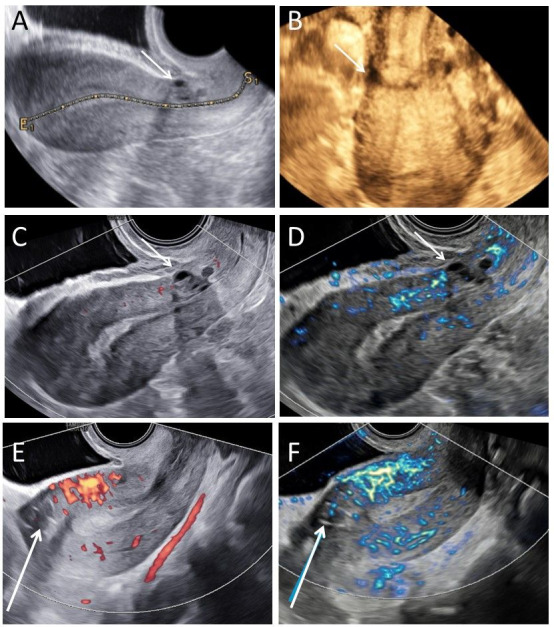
Representative images of pre- and postoperative assessment of scar tissue. A. Representative images of a complex niche shown in B-mode, sagittal view; B. Complex niche shown in three-Dimensional, coronal view; C. Complex niche shown in power Doppler mode; D. Complex niche shown in MV-flow^TM^ mode. Arrow indicates location of the complex niche and scarring tissue without vascularisation. E. Power Doppler image of the uterus 6 weeks after a laparoscopic myomectomy; F. Microvascular flow imaging after laparoscopic myomectomy, showing a hypovascularised area at the site of the incision, and clear flow at the proximal site. Arrow indicates stitches.
